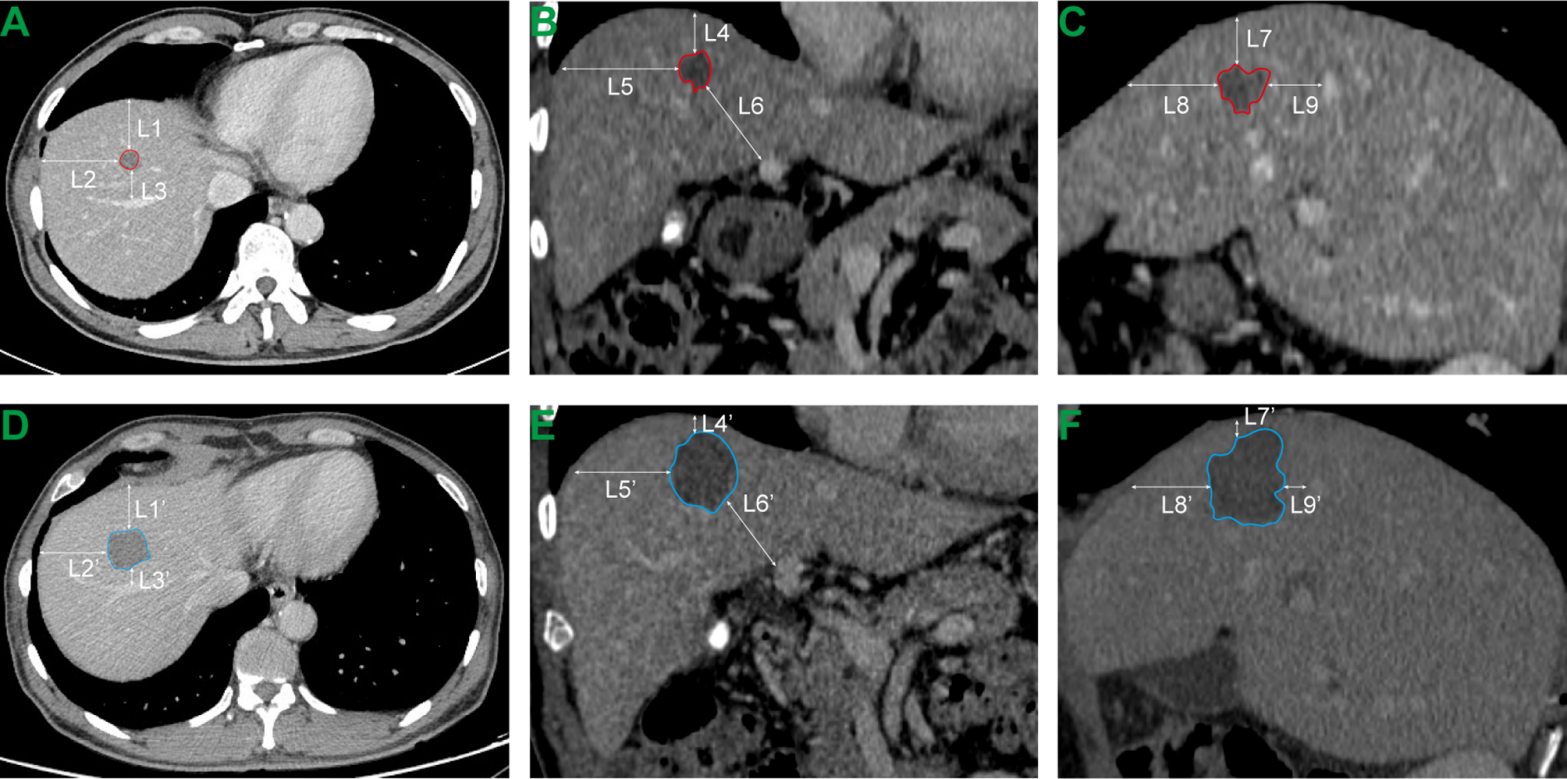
Measurement of minimal ablative margin. (A–C) Transverse, coronal and sagittal CT images before RFA. L1, L2,…, L9 respectively represent the distance from the tumor boundary to the selected anatomical landmark point. (D–F) Transverse, coronal and sagittal CT images of the same plane after RFA. L1’, L2’,…, L9’ respectively represent the distance from the perfusion defect zone generated by thermal ablation to the selected anatomical landmark point. The minimal ablative margin of RFA procedure is the minimum value of (L1-L1’), (L2-L2’),…, (L8-L8’) and (L9-L9’).
